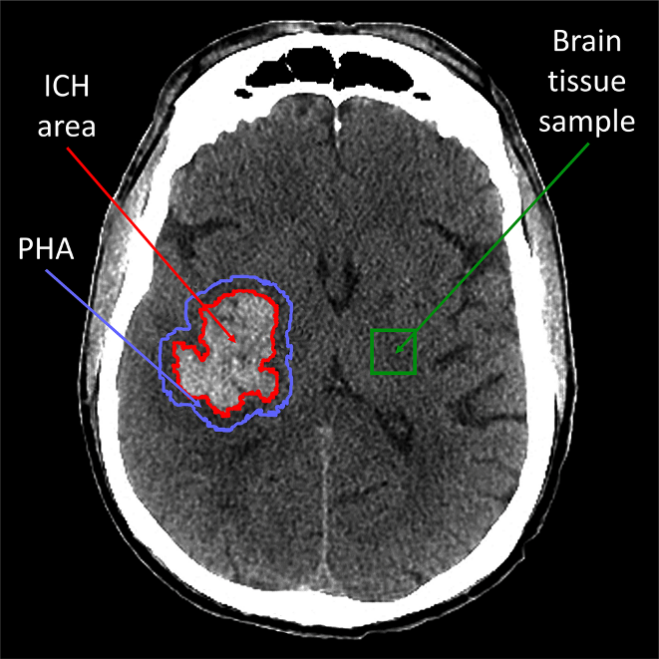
Exemplary axial head CT image segmentation. *Red contour* intracerebral hemorrhage contour with intracerebral hemorrhage image inside; area between *blue and red contour* perihemorrhage area image; area inside *green square* brain tissue sample image. *ICH* intracerebral hemorrhage, *PHA* perihemorrhage area
